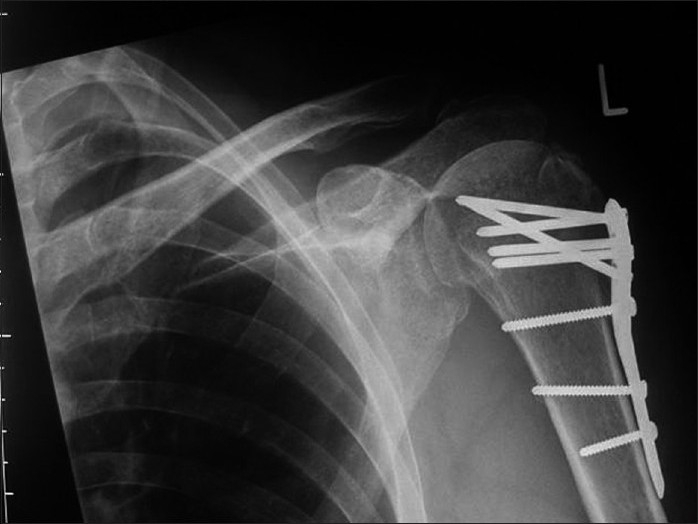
Note the multiple angled screw fixation and solid bony union evident on the postoperative radiograph at eight months, with no signs of avascular necrosis
